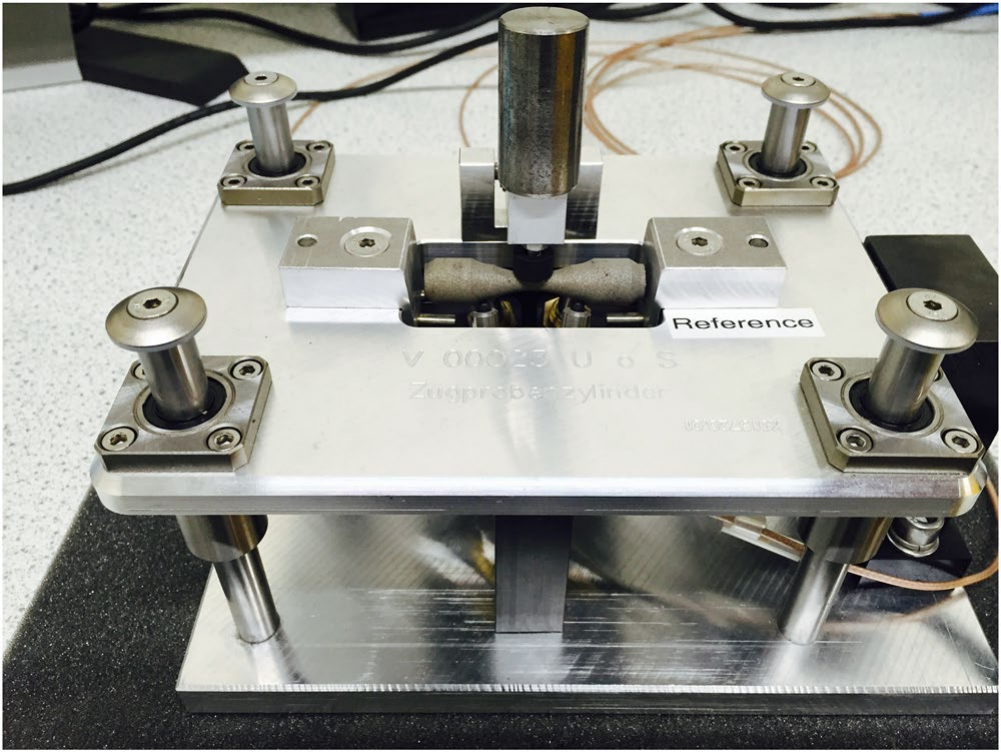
Resonance ultrasound testing jig.
